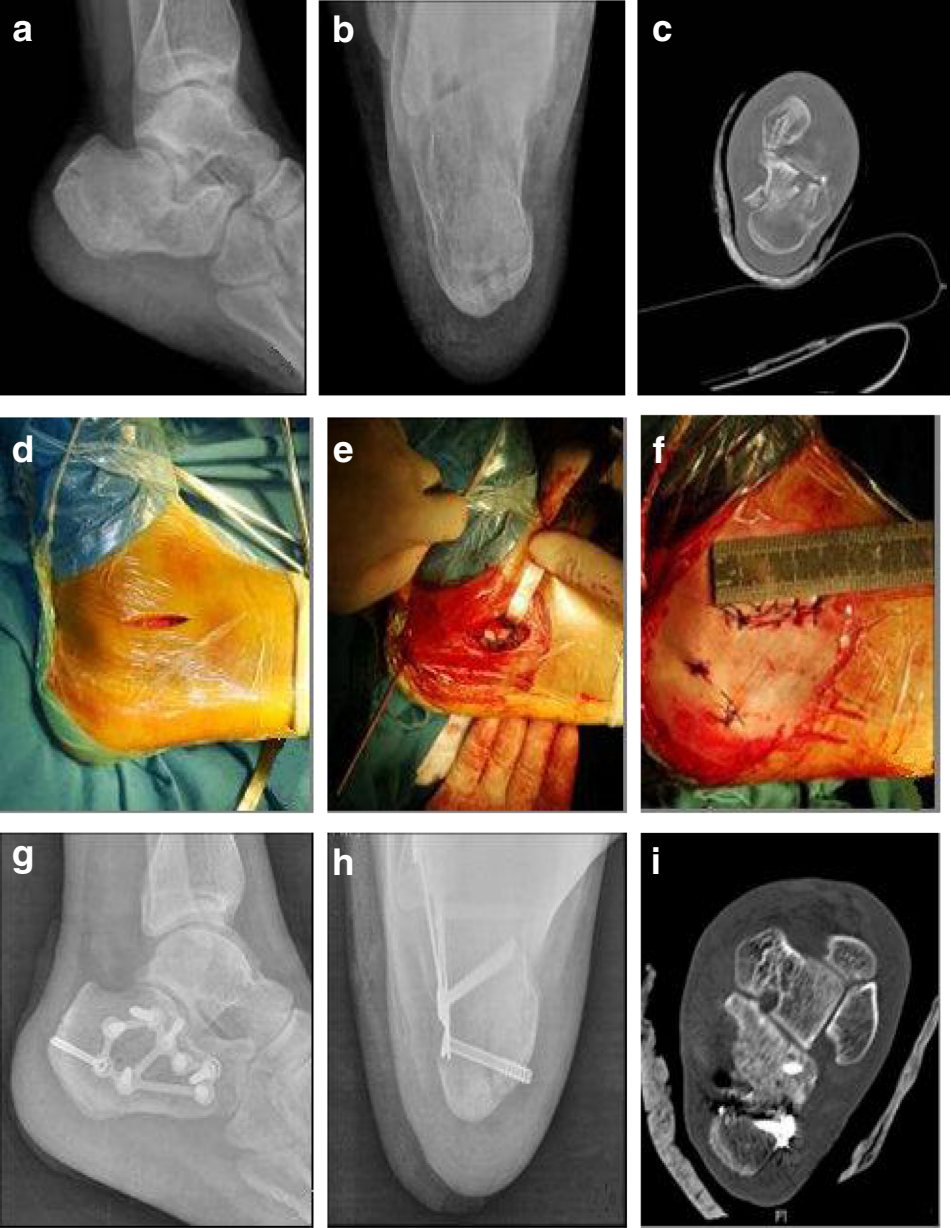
A 38-year-old male patient suffered right lateral displaced intra-articular calcaneal fracture caused by a fall from a height. Preoperative X-ray: lateral view (a) and axial view (b) showed the calcaneal height/length, Bohler angle, and the Gissanes angle was significantly decreased, and the calcaneal width was significantly increased. Preoperative CT-scan (c) showed a Sanders Type-IIIAB calcaneal fracture with collapse of the subtalar articular surface. Intraoperative incision showed a 4.0 cm minimally invasive sinus tarsi approach (d), the anatomical plate was placed through the subcutaneous tunnel (e), and the incision was sutured (f). Postoperative X-ray: lateral view (g) and axial view (h) showed calcaneal anatomical reduction. Postoperative CT-scan (i) showed anatomic reduction of the articular surface and restoration of the calcaneal width
